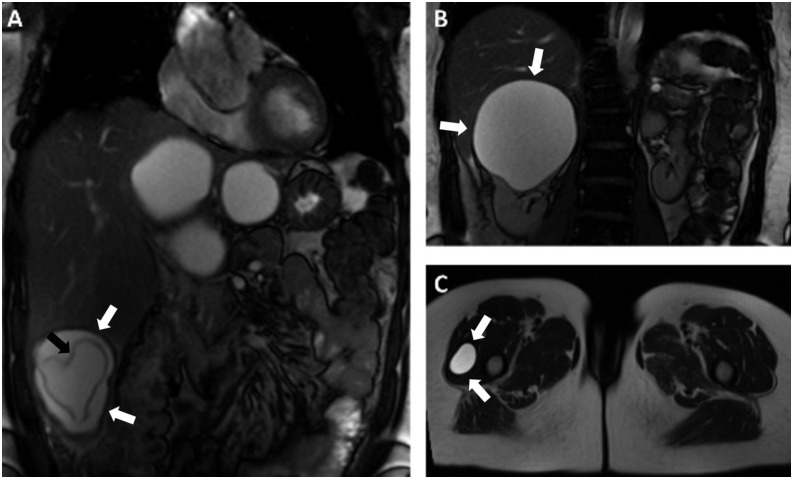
Coronal (A and B) and axial (C) T2-weighted images show hepatic, renal, and muscular hydatid cysts (white arrows). Note that a linear hypointense structure resembling an inner membrane is seen in the hepatic cyst (black arrow).
